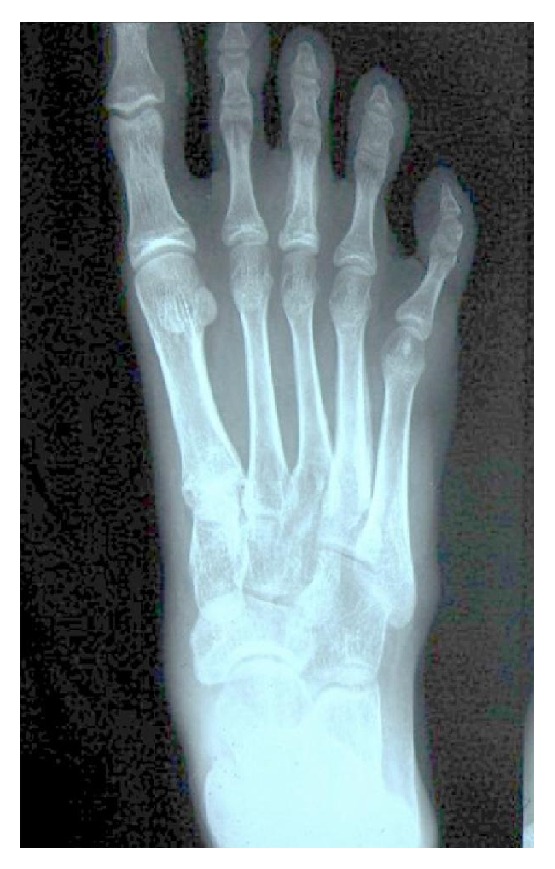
Radiograph of right foot (AP view), showing irregular destruction of proximal phalanx of 3rd toe and cortical thickening of 4th metatarsal with solid periosteal reaction.
